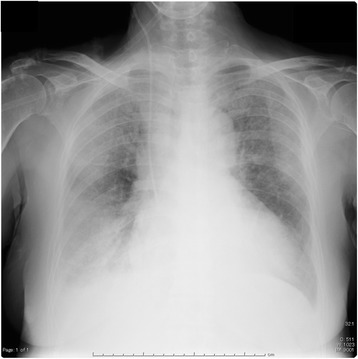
Chest radiograph indicated extensive bilateral infiltrates
